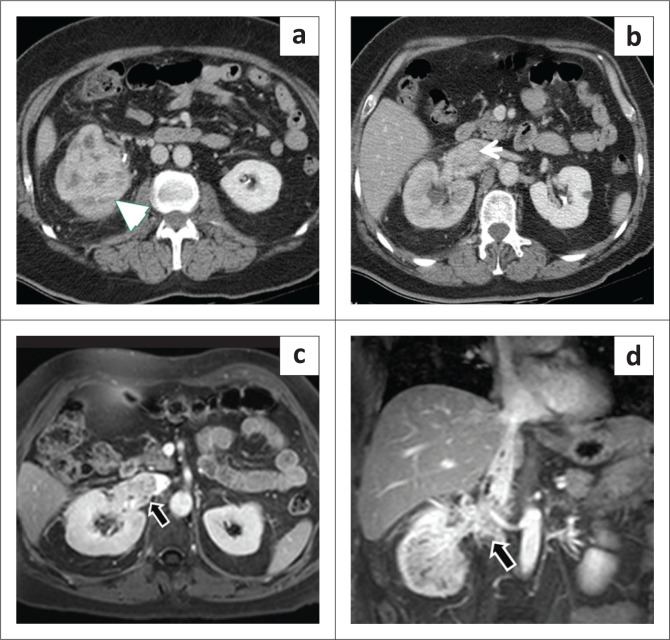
(a, b) The axial contrast-enhanced CT images demonstrated a heterogeneously enhancing mass (arrowhead) arising from the right kidney, which was proven to be renal cell carcinoma with an enhancing filling defect (thin white arrow) in the inferior vena cava. (c, d) The axial and coronal post-contrast T1-weighted fat saturated images revealed enhancement within the filling defect suggestive of tumour thrombus (black arrow).
